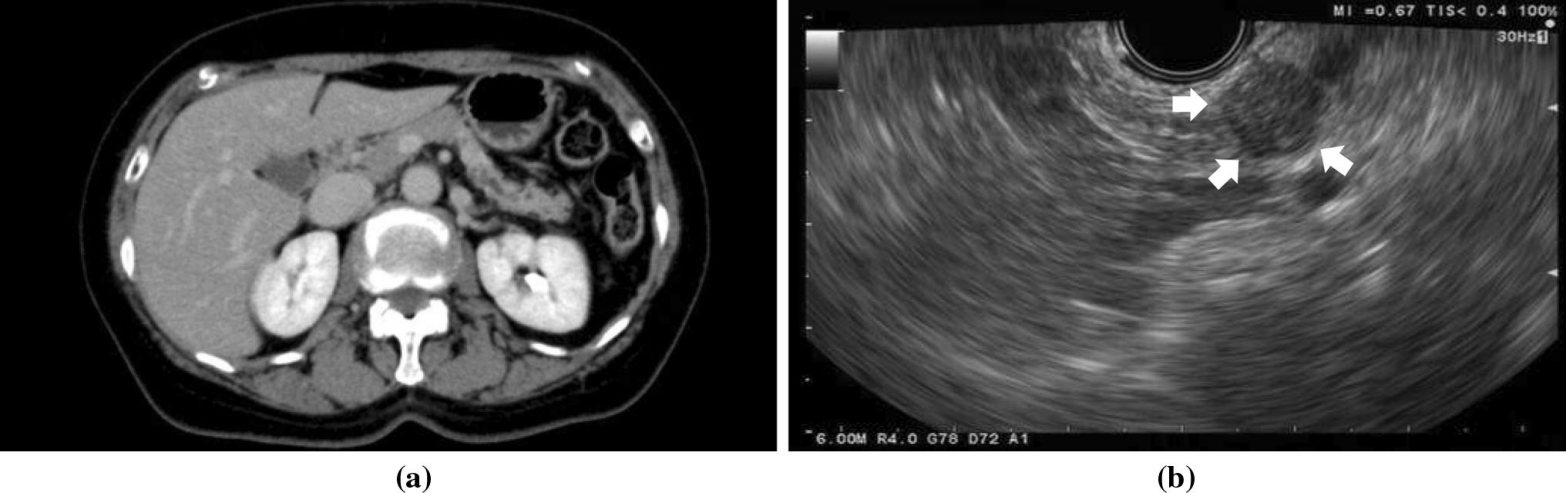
A case of small ductal carcinoma (8 mm, pancreatic body). Pancreatic mass was not detected by enhanced contrast MDCT (a), whereas detected clearly by endoscopic ultrasound (b, arrowheads)
